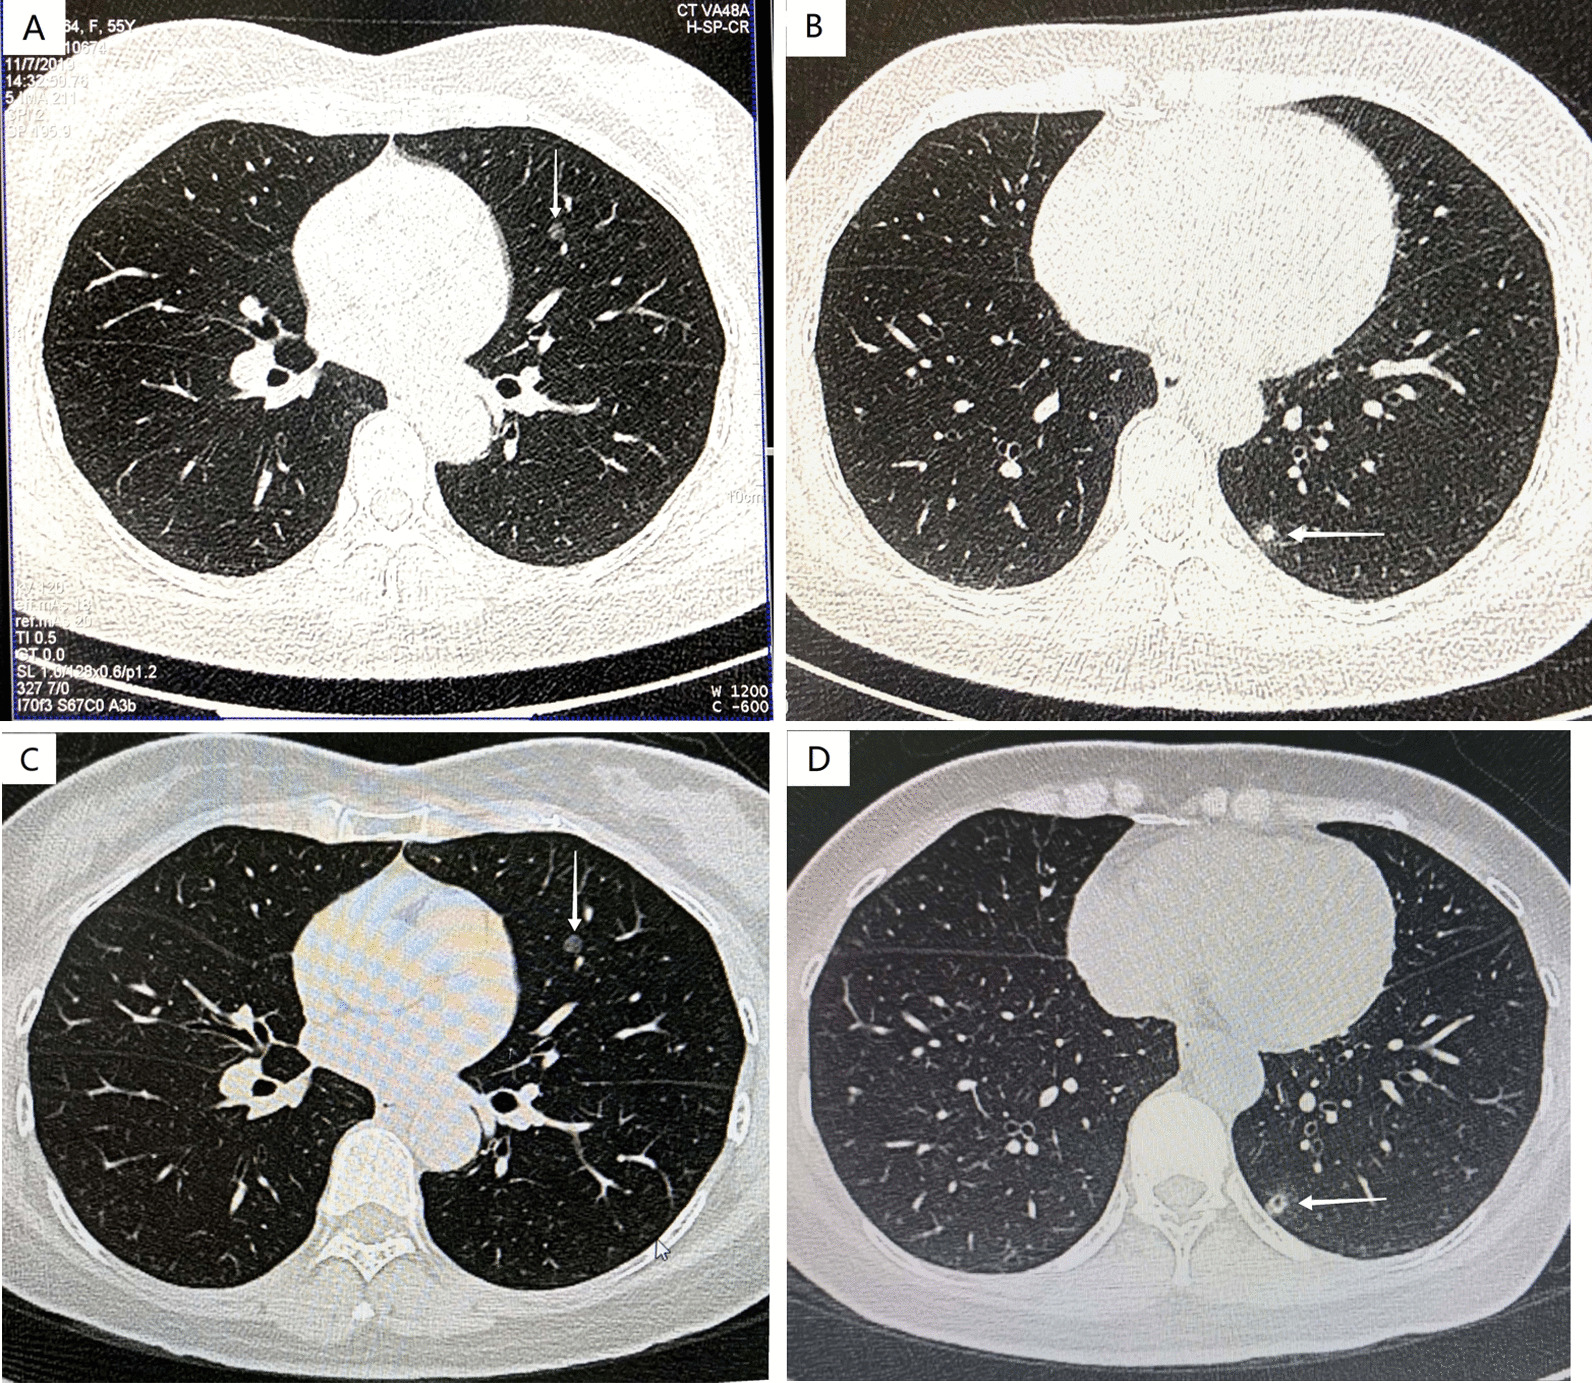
Computed tomography (CT) findings of BAs (arrows). a and c: a ~ 6 mm round pure GGN peripherally located in the lingual segment (a), and almost no change one year later (c). b and d a ~ 7 mm mixed GGN with irregular ill-defined peripheral opacity located in the posterior basal segment, adjacent to pleura (b), and slightly enlarged to 8 mm in diameter with vacuole sign one year later (d)
